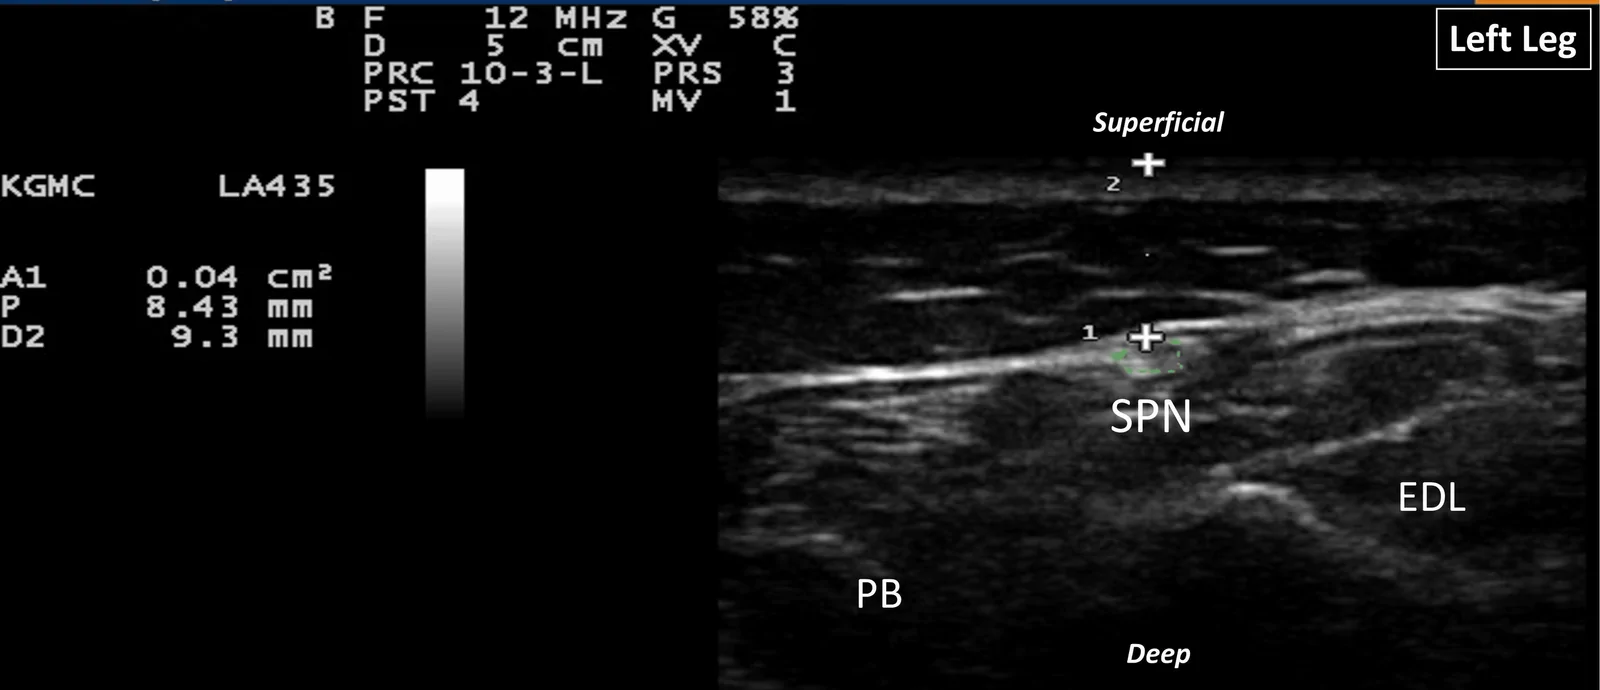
Ultrasonographic image of left leg showing the superficial peroneal nerve before piercing the deep fascia. EDL, extensor digitorum longus; PB, peroneus brevis; SPN, superficial peroneal nerve.
